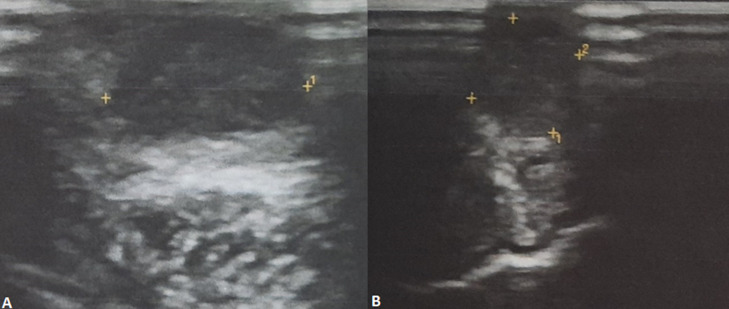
(A,B) ultrasonography examination showing a hypoechoic well-defined lesion with smooth margins within substance of the tongue
